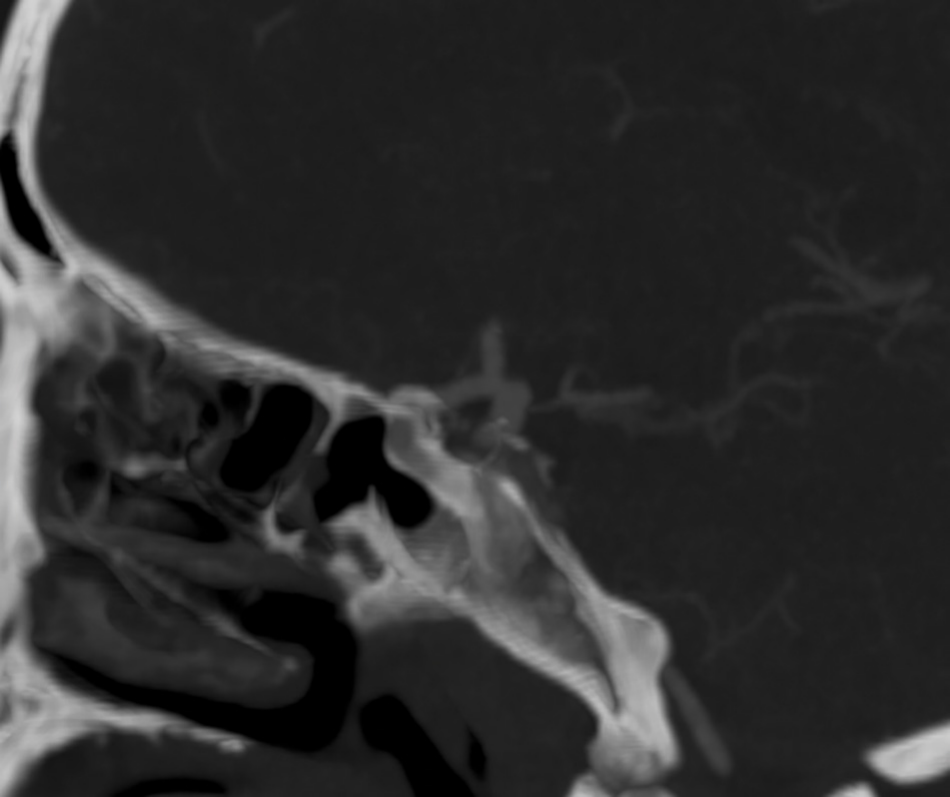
CT angiography revealing an irregular 10 mm aneurysm of the right posterior communicating artery.
